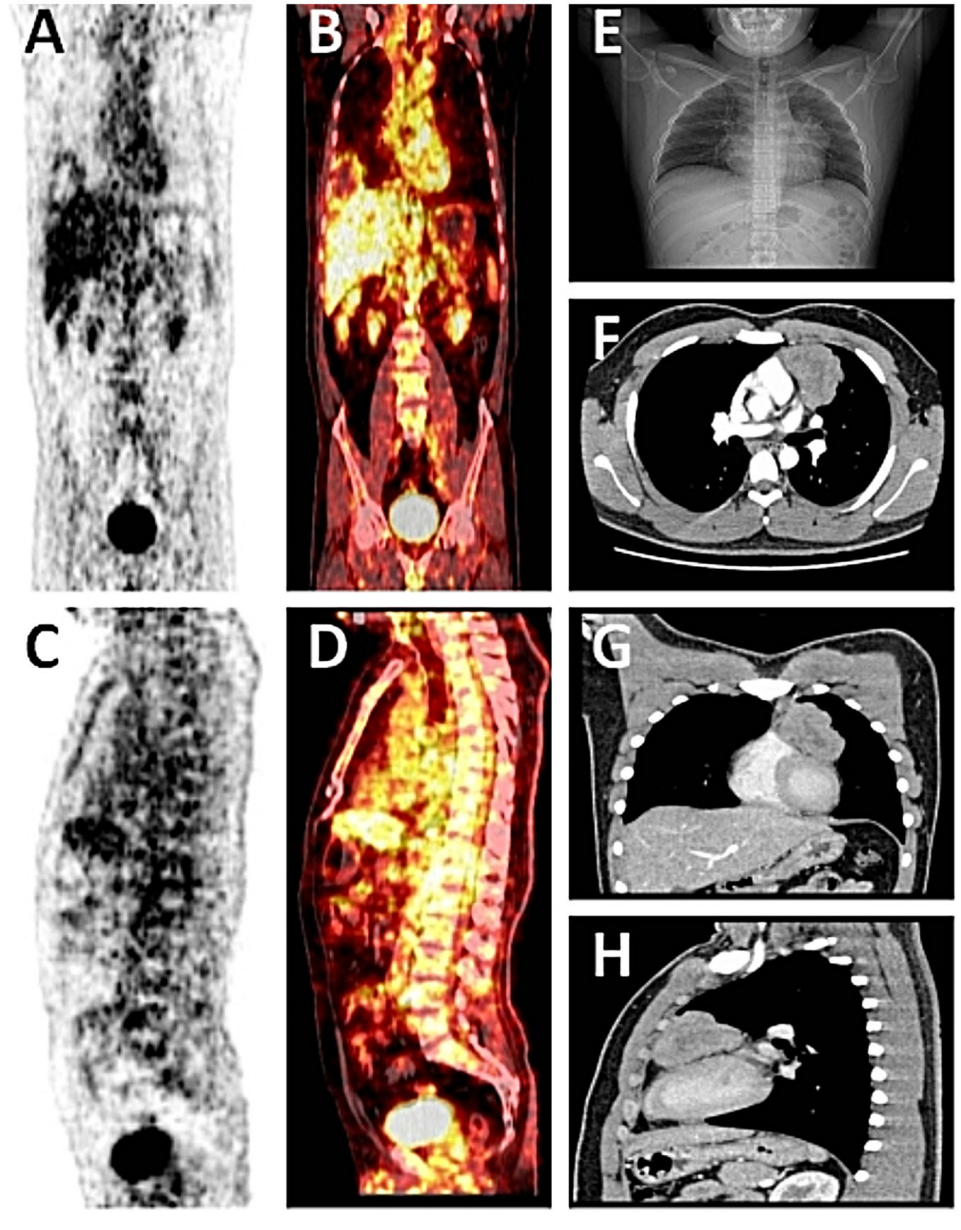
(A-D) Whole-body PET-CT showed a left anterior mediastinal soft tissue shadow, an uneven increase in radioactive uptake (SUVmax = 4.5), and no obvious abnormalities in other organ tissue examinations. (E) A chest X-ray showed a left anterior mediastinal tumor with illuminated edges. (F-H) Chest CT showed a large mass of soft tissue density in the left anterior upper mediastinum, with lobulated edges and uneven internal density, and mild to moderate enhancement of the solid part of the edge on enhanced scanning.
